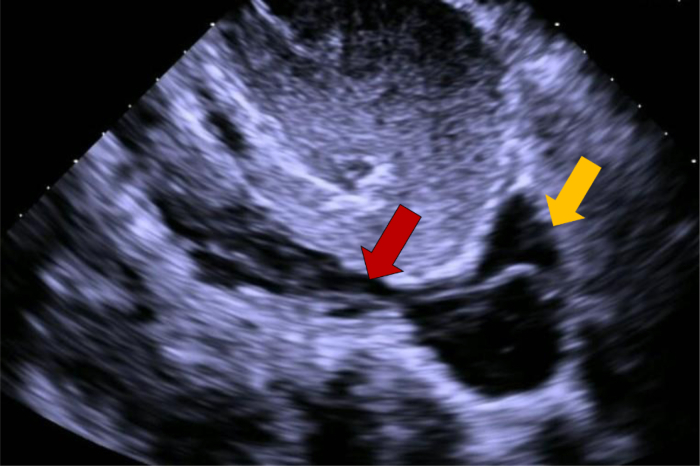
Transthoracic Echocardiography Findings TTE reveals a mobile echogenic mass within the right atrium, which intermittently prolapses toward the tricuspid valve during diastole. The red arrow highlights a tubular structure extending from the IVC into the atrial chamber, consistent with a tumor thrombus or an extension of intravascular leiomyomatosis. The yellow arrow indicates the right atrial cavity. Additional dynamic imaging is provided in Video 1, Video 2, Video 3, Video 4. IVC = inferior vena cava; RA = right atrium; TTE = transthoracic echocardiography.
